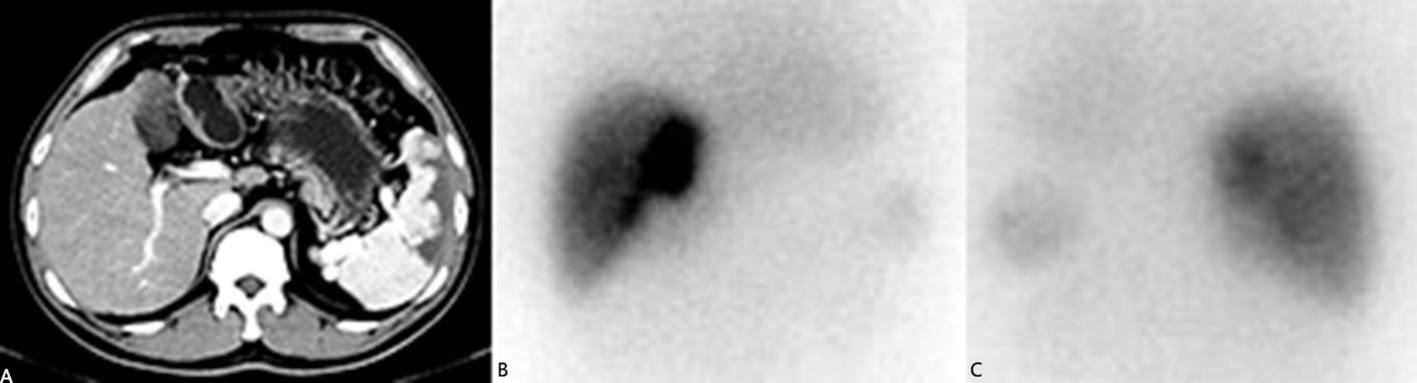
(A) The liver computed tomography suggested the postoperative stump recurrence of the right lobe (5.8 × 3.6 cm^2^). High density shadow of spleen could be seen. This patient had splenic artery embolism before due to hypersplenism; (B) The anterior image of the hepatic area in 131-I whole-body scanning revealed increased 131-I uptake in recurrent tumors; (C) The posterior image of the hepatic area in 131-I whole-body scanning revealed increased 131-I uptake in recurrent tumors.
